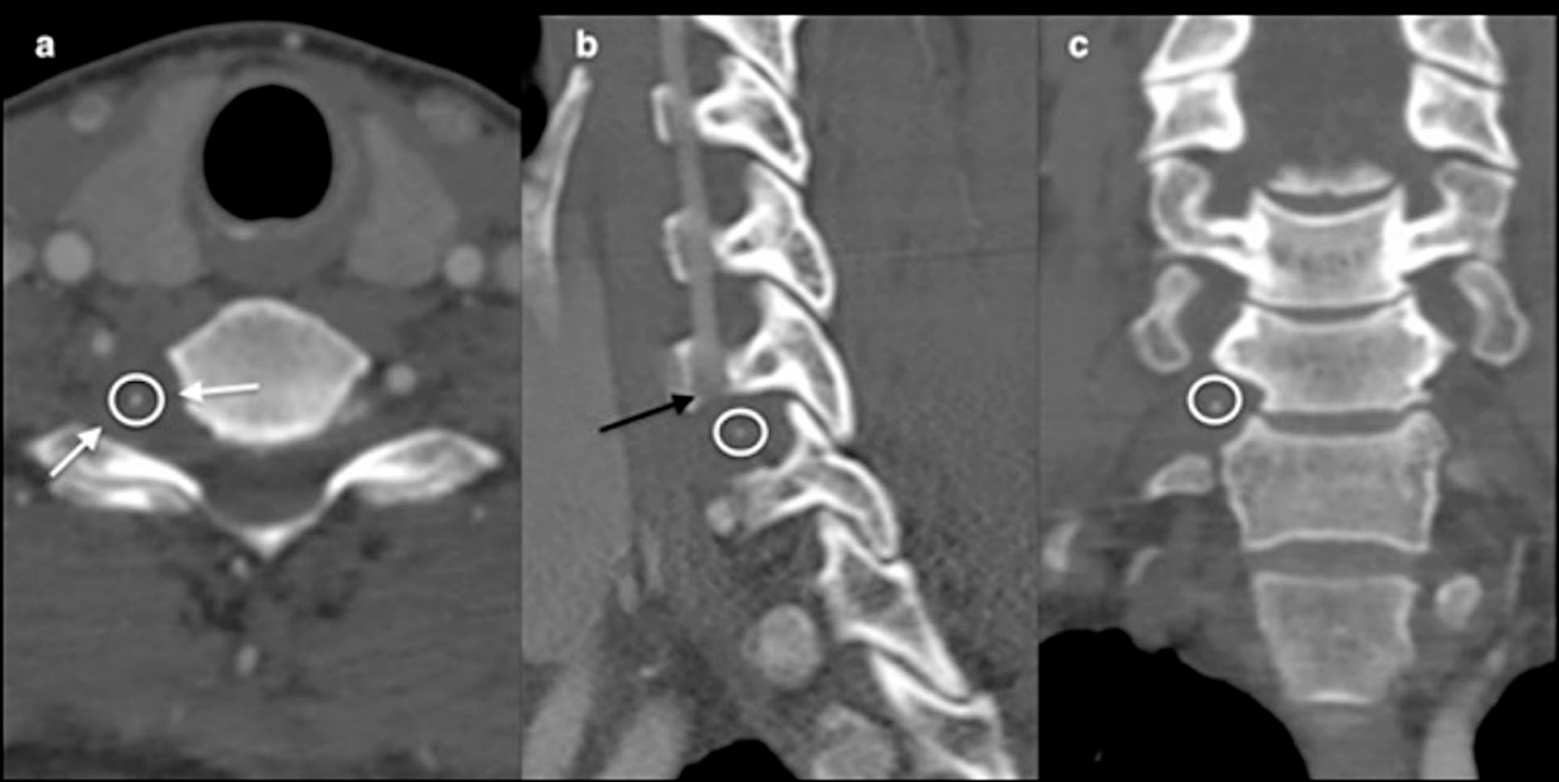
Cervical CT shows a right C6-C7 foraminal lesion with intralesional calcifications (white circle). Panel a: axial images. Panel b: sagittal images, the black arrow shows a right C6-C7 foraminal mass in close relationship with the vertebral artery without arterial obstruction. Panel c: coronal images
